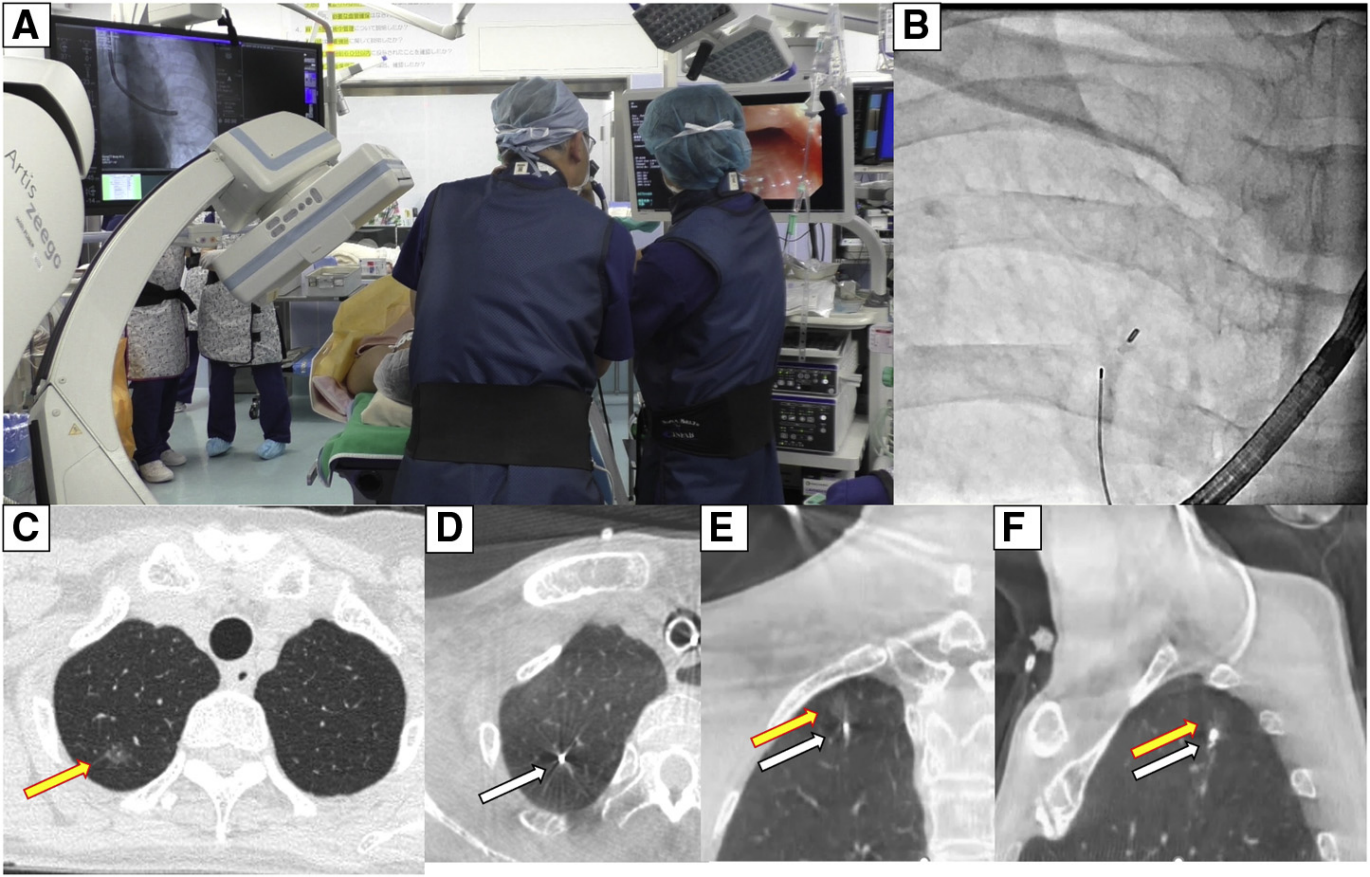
Radiofrequency identification (RFID) marking procedure using cone-beam computed tomography (CBCT) in a hybrid operation theater. A, RFID marker placement was conducted using CBCT in a hybrid operation theater just before surgery. B, Intraoperative fluoroscopy showing the RFID marker successfully placed in the airway. C, Preoperative computed tomography (CT) image showing a 5-mm lesion with pure ground-glass opacity located 15.3 mm from the pleura. D-F, Post-RFID-marking CT image showing the marker placed near the target with no apparent adverse effects (transverse, coronal, and sagittal planes, respectively).
